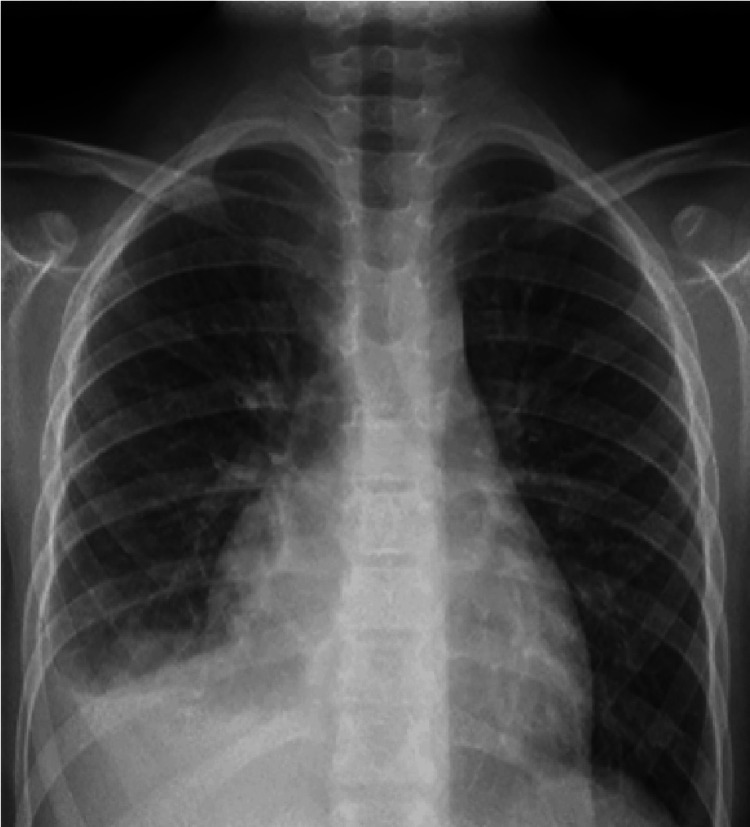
Chest radiograph showing mild cardiomegaly and right pleural effusion
